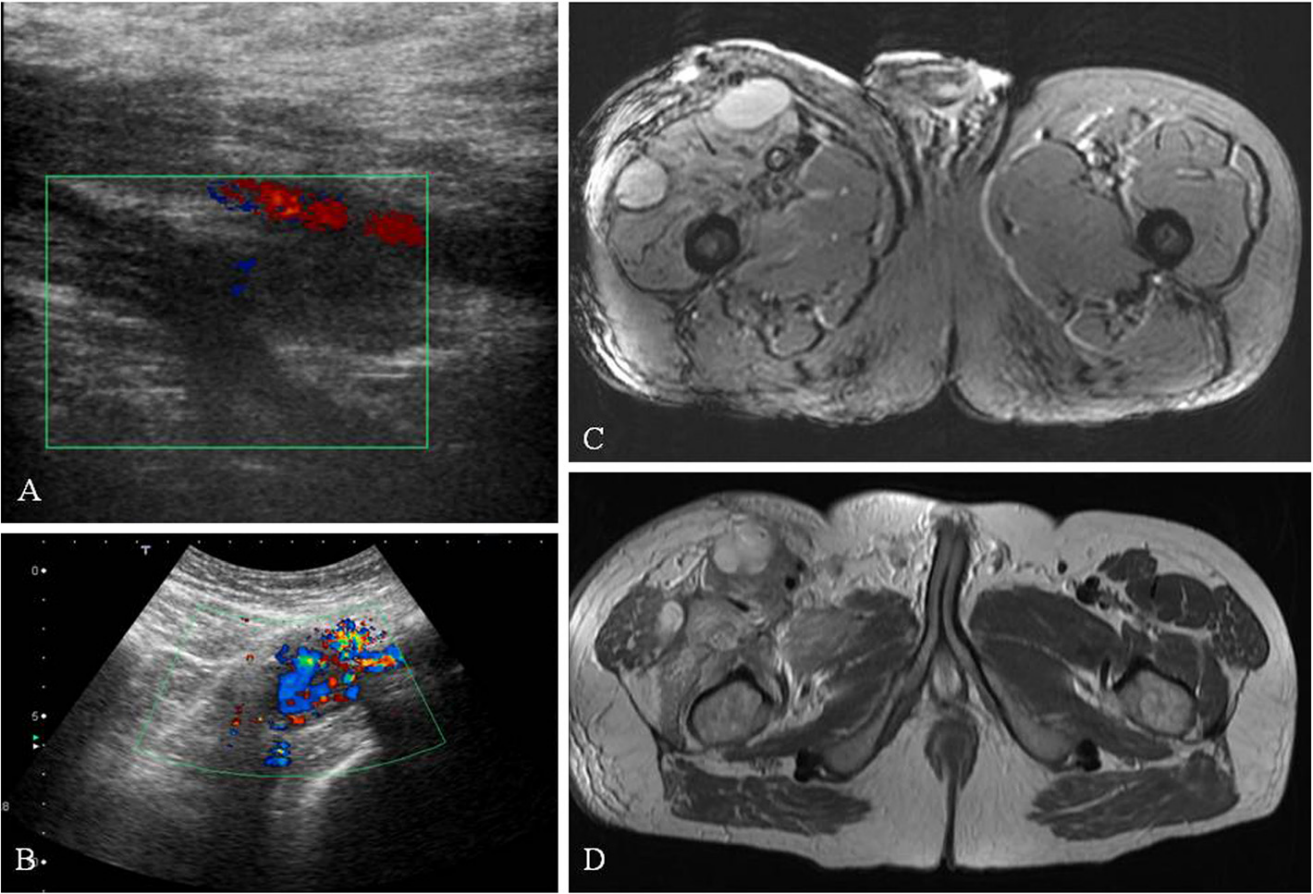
A,B: Doppler ultrasonography showing thrombosis of the right femoral and iliac vein with artero-venous microfistulas.C,D: MRI of the femur showing a leiomyosarcoma with thrombosis of the superficial and common femoral veins and involvement of the internal iliac vein.
